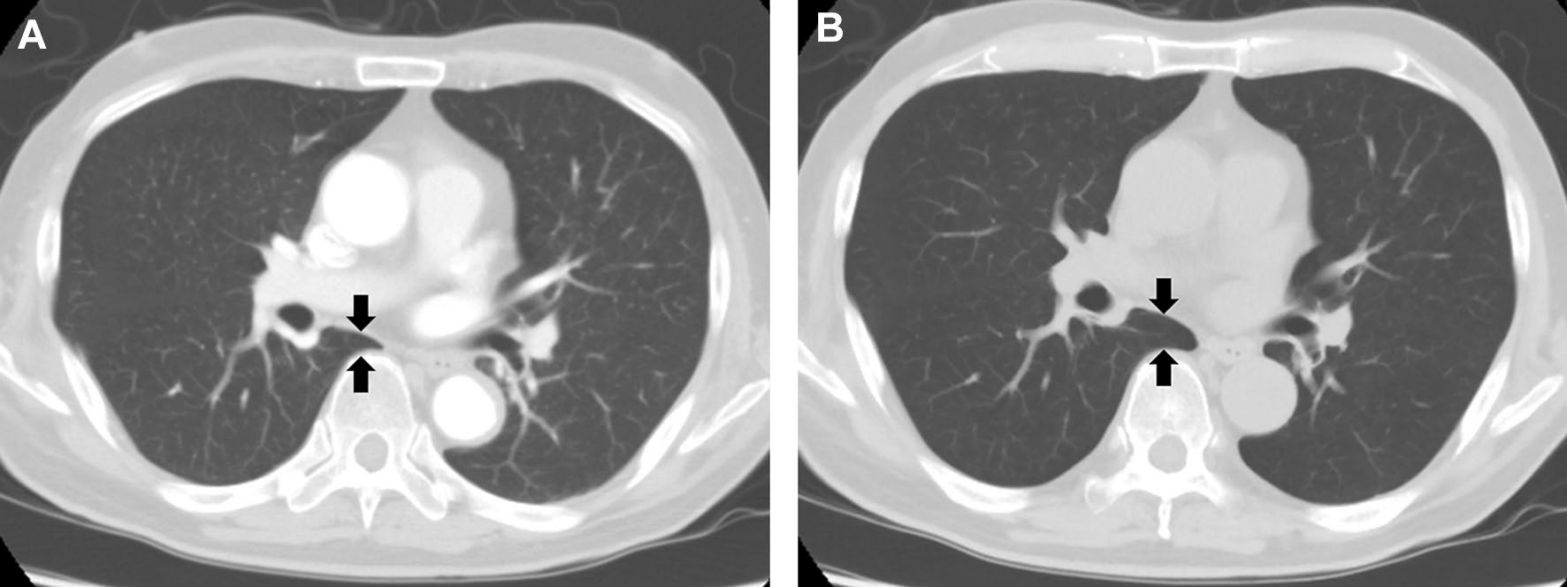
CT images of a 73-year-old male patient who was injected with iopamidol at 300 mgI/ml from the right antecubital vein. This patient underwent both the CT pulmonary angiography and unenhanced CT on the same day. Tracheal diameter/both lung volume was 19.0 mm/3943 ml in CT pulmonary angiography A and 21.0 mm/4807 ml in unenhanced CT. B Reduction of lung volume can be visually recognized with the reduction of anteroposterior diameter of the azygoesophageal recess (black arrows)
